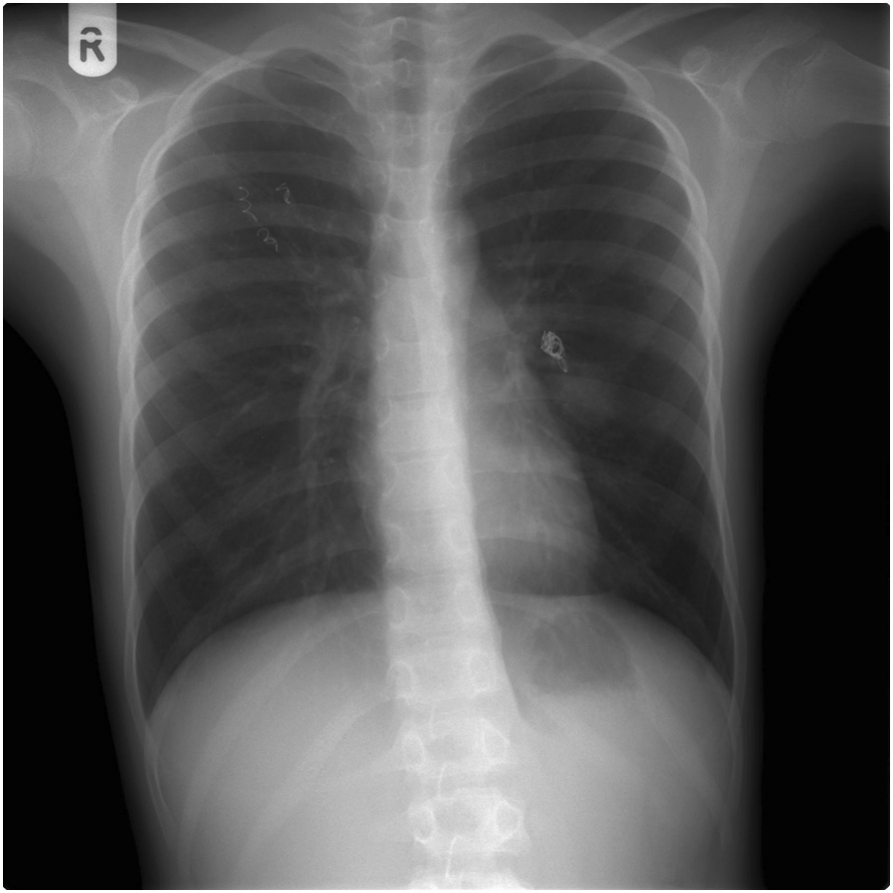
Chest X-ray with visible steels coils after embolotherapy of pulmonary arteriovenous malformations within the perihilar left upper lobe and small arteriovenous malformation within the apical segment of the right lobe.
